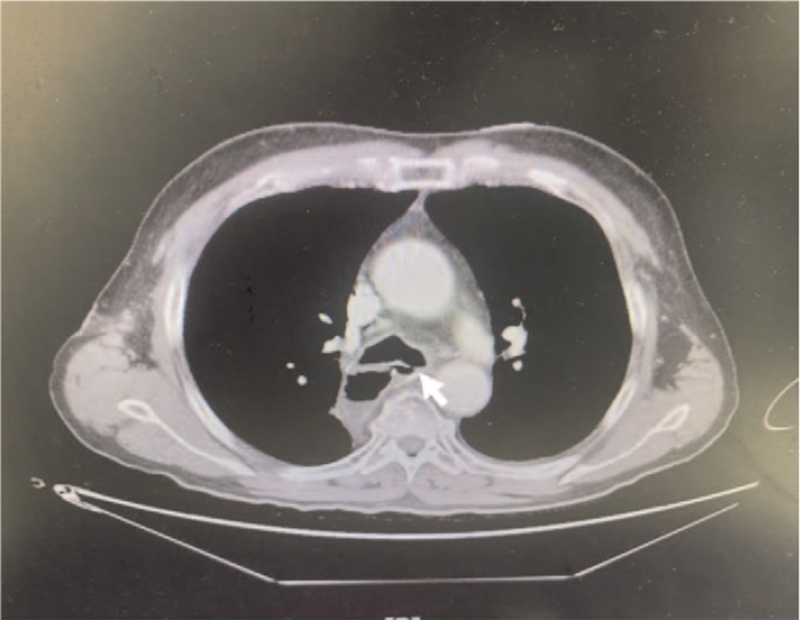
Computed tomography of the chest shows a fistula between the left main bronchus and the gastric conduit, indicated by a white arrow at the level of the carina.
